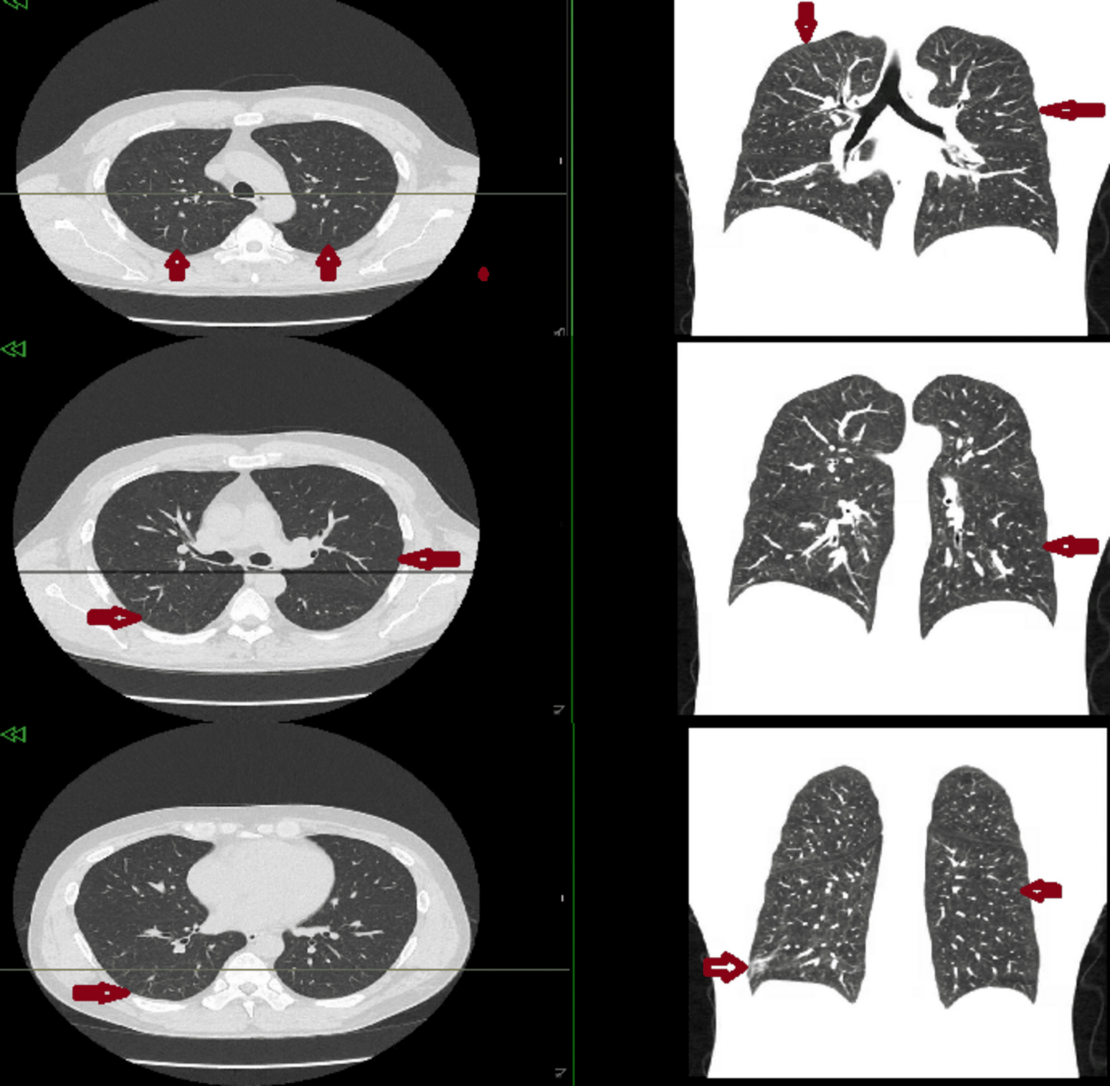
Axial and coronal views of chest CT scan without contrast at three different levels, highlighting interval resolution Red arrows mark the same previously involved areas for comparison.
